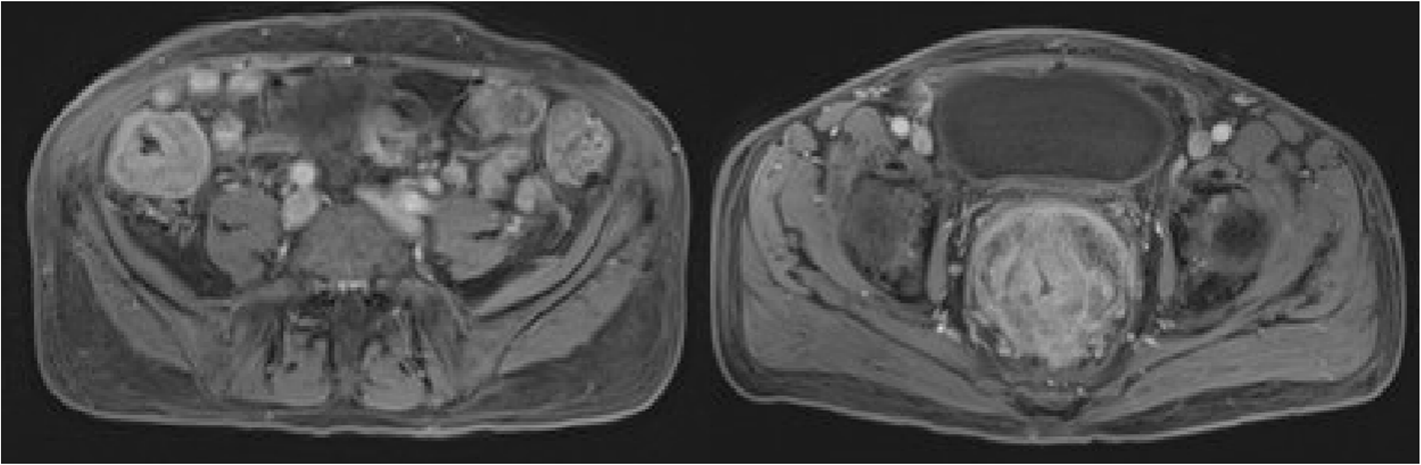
Magnetic Resonance Imaging scan showing circumferential thickening of cecum and rectum pre treatment.
